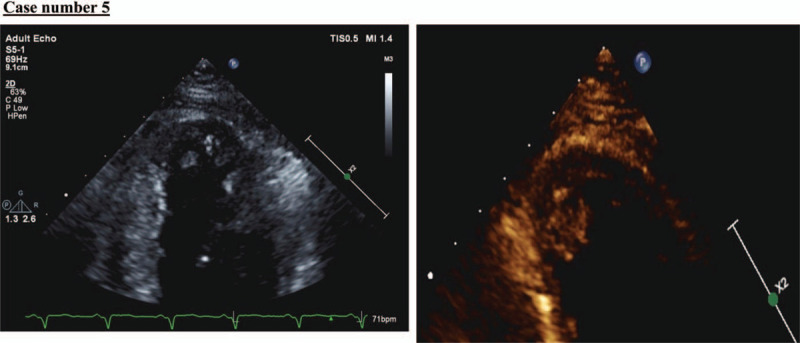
Echocardiography showing a left ventricle with a moderate sized (14 mm × 14 mm) apical thrombus.
